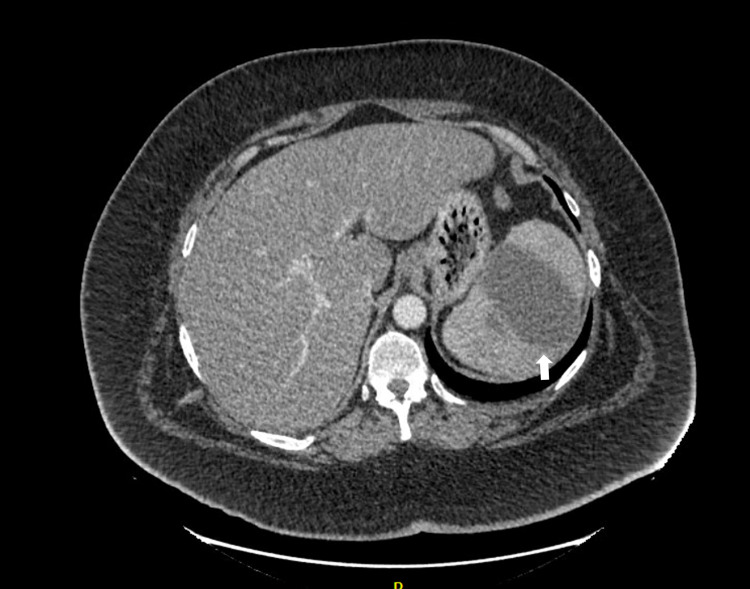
Splenic infarction (arrow) seen on arterial and PV phase CT scan PV, Portal vein; CT, computed tomography.
